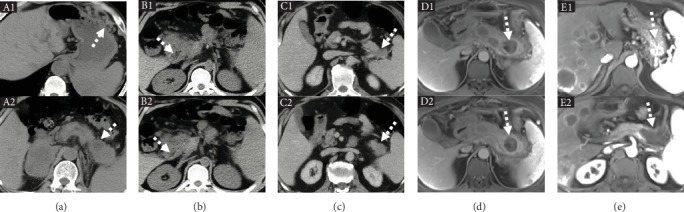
Images regarding pancreatic lesions in five LPSH patients. (a) Plain abdominal CT of case 1 (TL) demonstrating multiple distorted soft issue mass densities in the left upper abdomen (A1, white dotted arrow) and a cystic lesion in the pancreatic tail (A2, white dotted arrow). (b) Plain abdominal CT of case 2 (YC) demonstrating a distorted pancreatic head with local hypodensity (B1 and B2, white dotted arrows). (c) Contrast-enhanced abdominal CT of case 3 (WZ) demonstrating a mass in the pancreatic tail (C1 and C2, white dotted arrows). (d) Contrast-enhanced abdominal MR of case 4 (JH) demonstrating acute hemorrhagic necrotizing pancreatitis with pancreatic pseudocyst (D1 and D2, white dotted arrows). (e) Contrast-enhanced abdominal MR of case 5 (HW) demonstrating multiple gastric and splenic varices (E1, white dotted arrow) and pancreatic cystadenocarcinoma in the pancreatic tail (E2, white dotted arrow).
